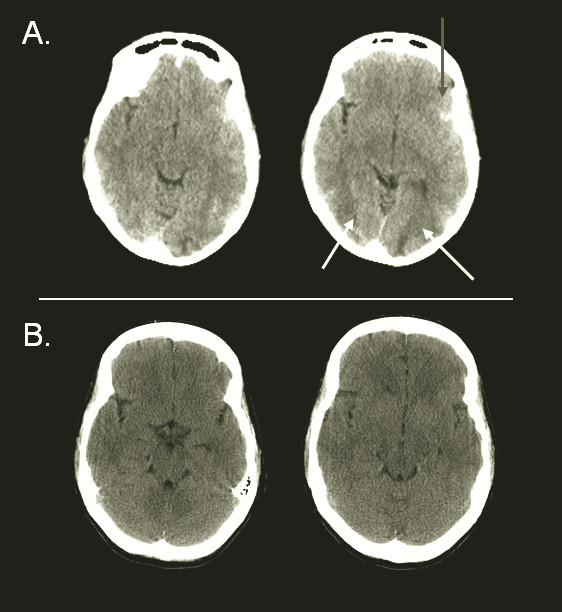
(A) Brain computed tomography (CT) performed at the time of presentation showed a small amount of blood in the left Sylvian fissure (gray arrow) and bilateral edema in the occipital regions (white arrows), suggestive of posterior reversible encephalopathy syndrome. (B) Brain CT performed 10 days later shows a total resolution of radiological signs.
